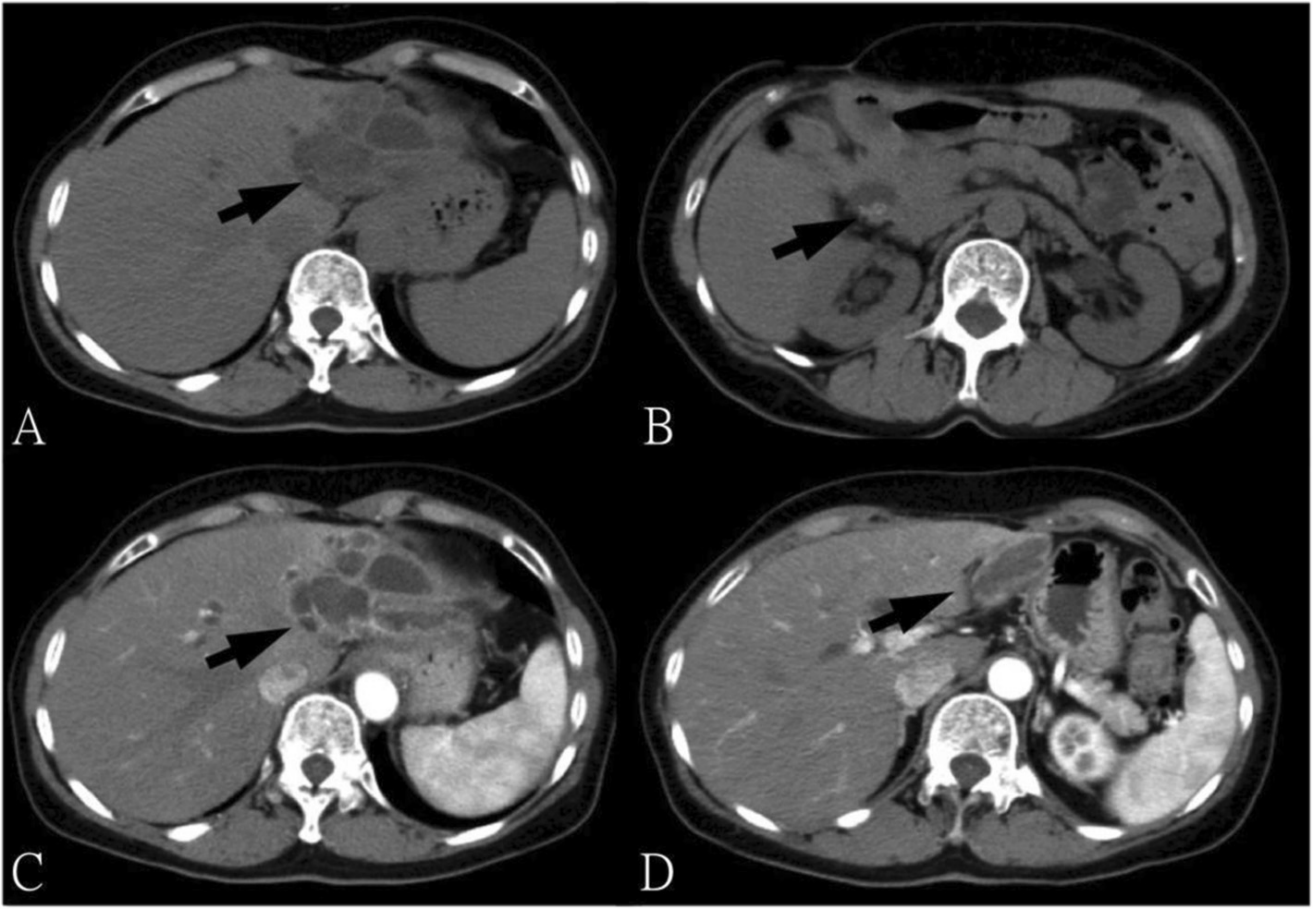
Computed tomography of the liver and bile duct. (A) Computed tomography (CT) disclosed multiloculated cystic lesion with internal septa (arrow). (B) Gallbladder stone was identified (arrow). (C) After the administration of contrast medium, there was enhancement delineating the contour of the cystic tumor (arrow). (D) The cystic lesion extended to the lateral division of the left hepatic lobe (arrow).
